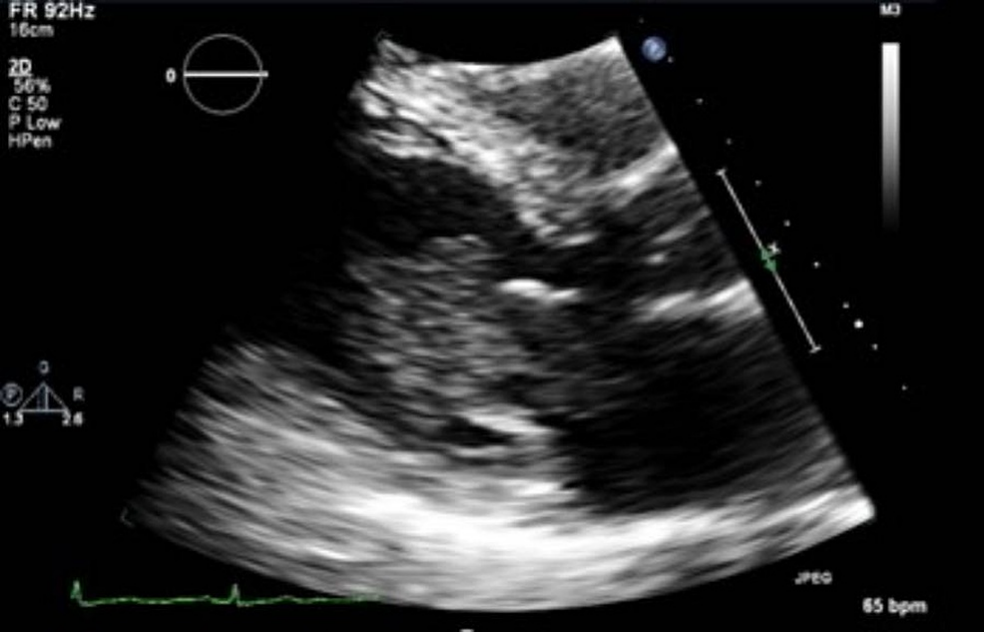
Echocardiogram showing ejection fraction >55%, enormous left atrial myxoma, and trace mitral regurgitation
